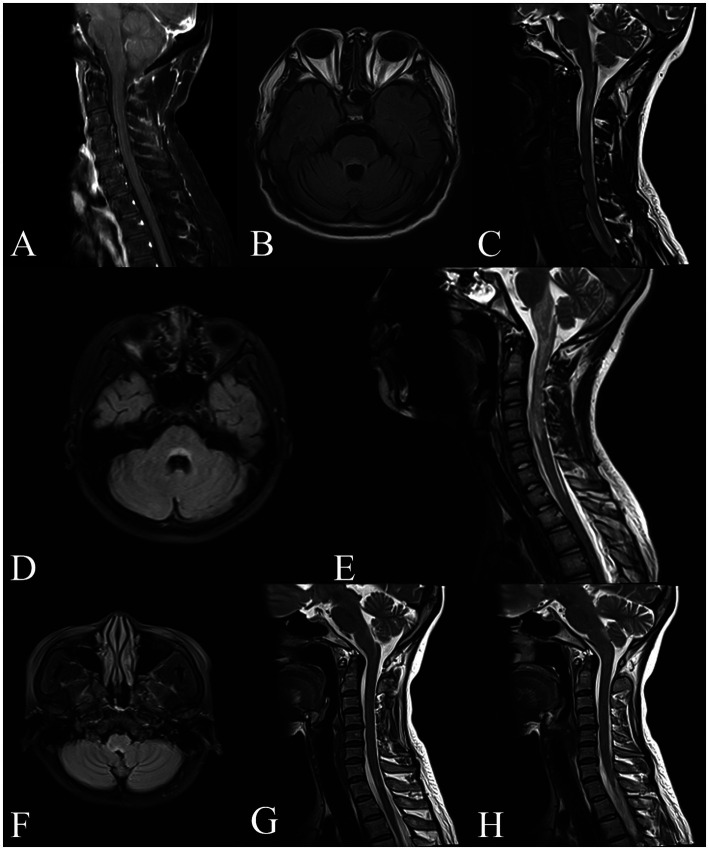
Brain and spinal cord MRI of GFAP-IgG positive patients with APS. Patient 1, (A) Sagittal T1-weighted MRI with gadolinium shows linear enhancement along the surface of the brainstem and in cervical spinal cord. Patient 3, (B) Axial T2-FLAIR imaging shows the lesion around the fourth ventricle. Patient 4, (C) Sagittal T2-weighted imaging shows a linear lesion in the medulla oblongata and upper cervical cord. Patient 5, (D,E) Axial T2-FLAIR imaging shows the “inverted V” lesion in the dorsal medulla oblongata involving the area postrema. Sagittal T2-weighted imaging shows a longitudinally extensive patchy lesion in the upper spinal cord extending to medulla oblongata. Patient 6, (F,G,H) Axial T2-FLAIR imaging shows the “inverted V” lesion in the dorsal medulla oblongata. Sagittal T2-weighted imaging shows the linear medullary lesion and the area postrema lesion.
